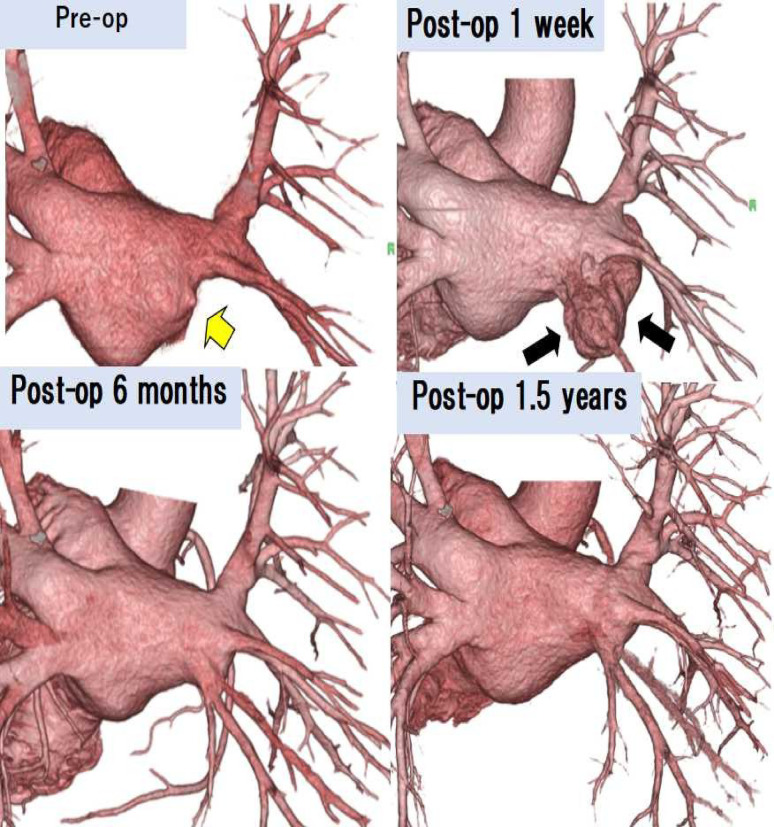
Three-dimensional cardiac computed tomography image (Case 4) : The yellow arrow indicates the site of pulmonary vein obstruction. An additional space (black arrows) involving the left atrial appendage was observed in the left atrium during the early postoperative period. Over time, the pulmonary vein appeared to form a direct anastomosis with the left atrium and gradually enlarged
